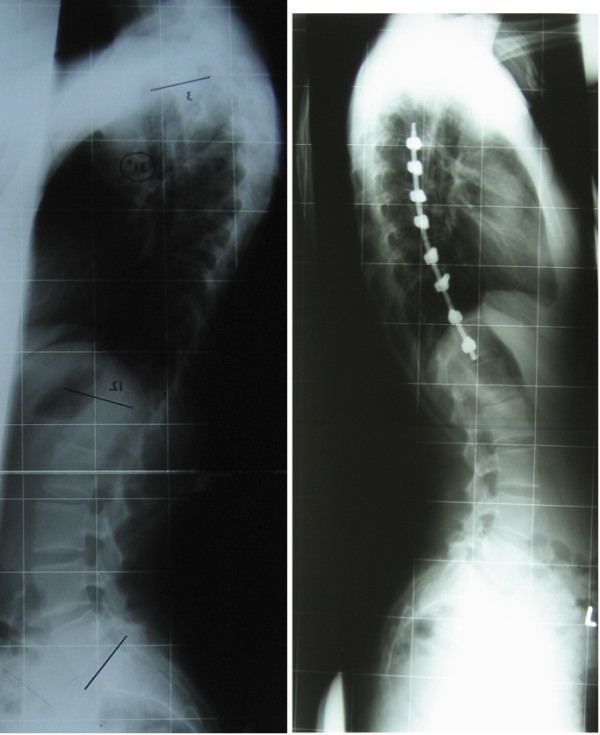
Preoperative curve of 69° on lateral radiograph (left) and postoperative curve on the right.
